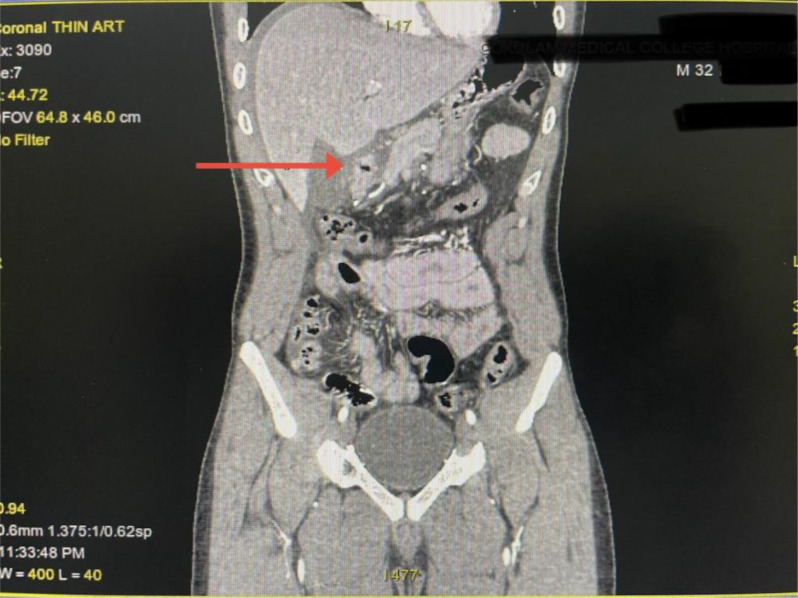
Coronal CT scan of Patient 2 demonstrating a gastric perforation (red arrow) with localized pneumoperitoneum
